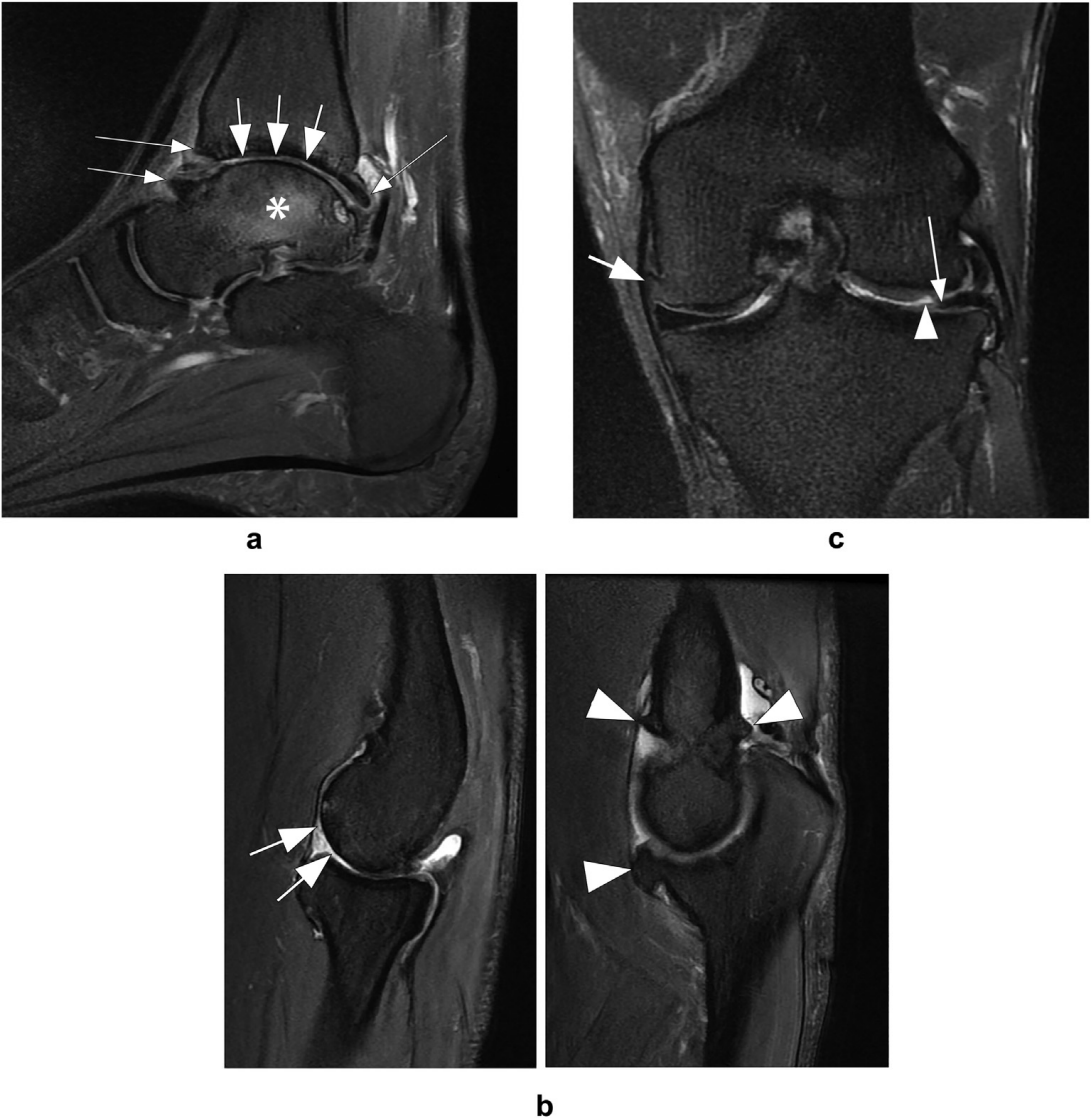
Example of different joints with osteoarthritis (OA) according to MRI assessment. A. Sagittal intermediate-weighted (IW) fat suppressed (fs) MRI of the ankle of a 34 year-old female athlete competing in track and field shows severe tibiotalar joint OA. There is diffuse wide-spread full thickness cartilage loss at the tibiotalar joint (short arrows). In addition there are definite anterior and posterior osteophytes (long arrows). Presence of these two features qualifies this joint for MRI-defined MRI-based OA. In addition there is marked subchondral bone marrow edema (asterisk) of the talus, a secondary feature of MRI OA. B. Sagittal IW fs images of the elbow of a 39 year-old male tennis player show diffuse full thickness cartilage loss at the capitellum humeri (arrows in the left image). In addition there are large marginal osteophytes (arrowheads in the right image). The combination of these two features qualifies this joint as having severe structural MRI OA. C. Coronal IW fs image of the knee in a 29 year-old weight lifter shows a definite marginal osteophyte at the medial femur (short arrow). In addition, there is a superficial focal cartilage defect (Outerbridge grade 2) at the lateral femur (arrowhead) and partial meniscal maceration of the free edge of the meniscal body (long arrow). Presence of these secondary features plus the presence of a definite osteophyte fulfills the criteria for a diagnosis of structural MRI OA. According to the definition used, this knee has mild OA.
